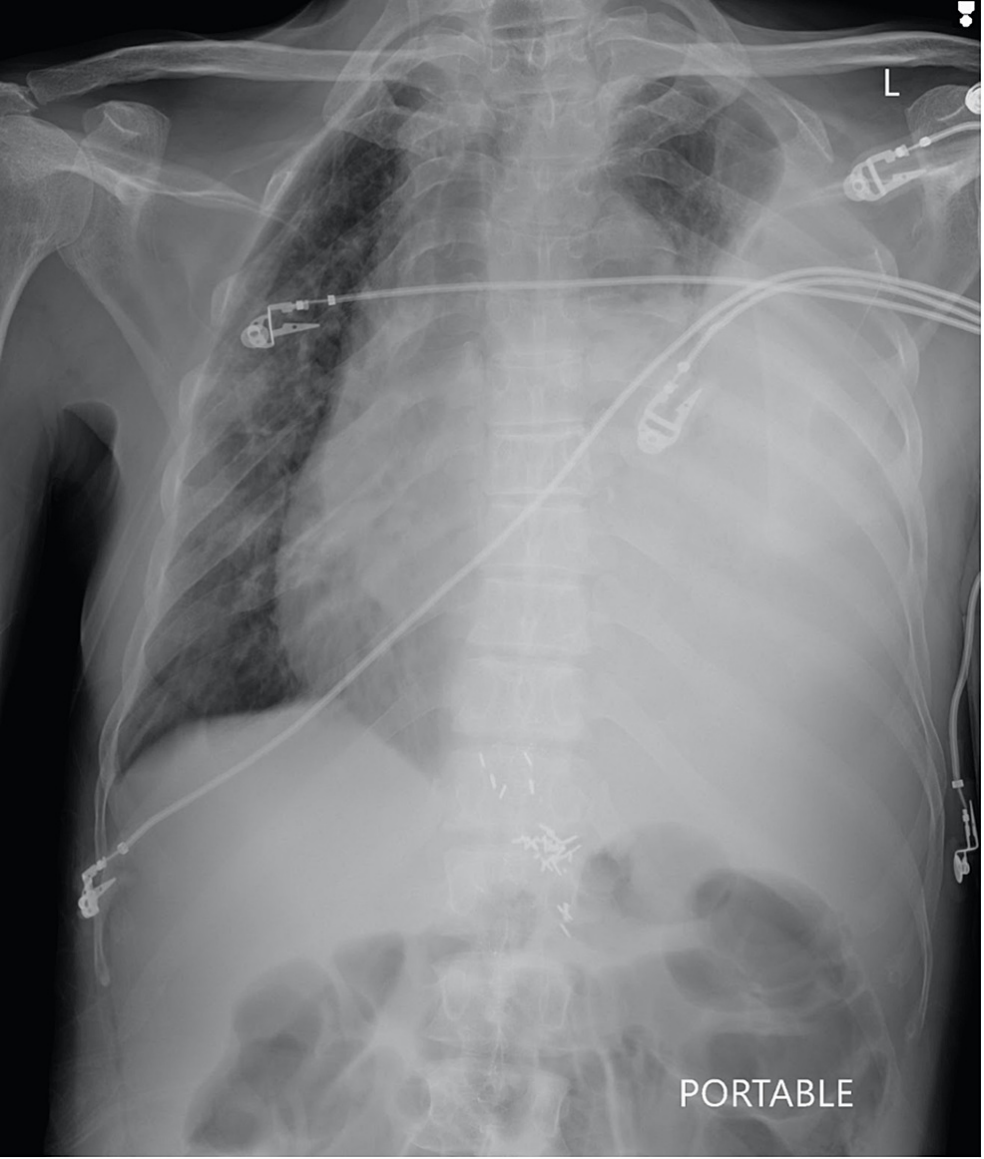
Chest x-ray demonstrating left-sided pleural effusion with rightward mediastinal shift consistent with a large-volume hydrothorax.
